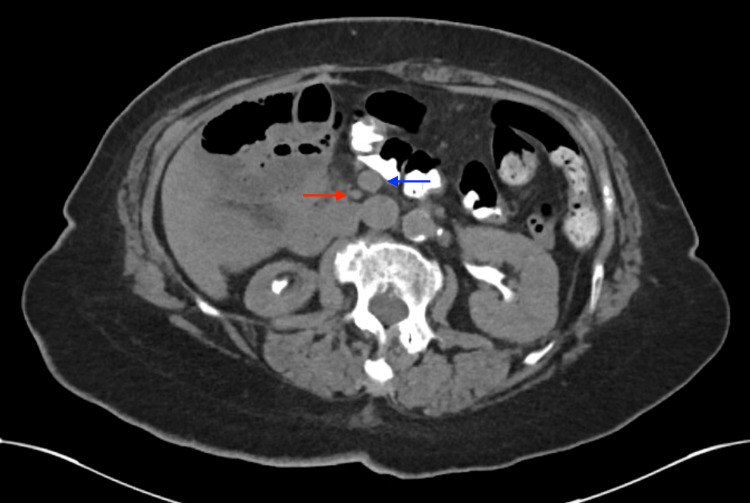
Axial CT abdomen showing the SMV (blue arrow) to the left of the SMA (red arrow), which is pathognomonic for intestinal nonrotation SMV - superior mesenteric vein; SMA - superior mesenteric artery
